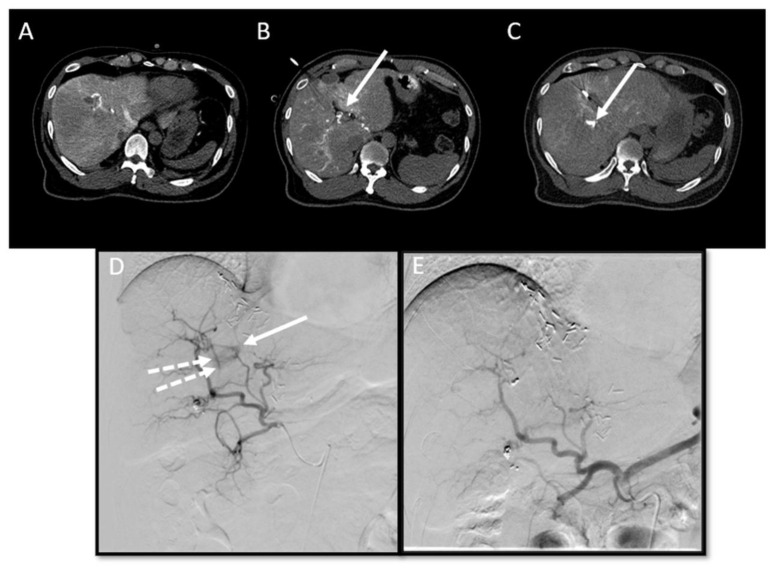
Critical care management: detection of post-ablation intraparenchymal hemorrhage and haemobilia. Probe-induced hepatic hemorrhage is seen in 0.7% of the patients [23]. Local tumor progression in segment 8 after parenchymal-sparing hepatectomy and intra-operative and percutaneous microwave ablations. After administration of 20 mL intra-arterial contrast (Visipaque™, iodixanol, 320 mg Iodine/mL, GE Healthcare Inc., Marlborough, MA, USA) in the common hepatic artery, the recurring tumor tissue became visible by a clear ‘incomplete ring sign’ adjacent to the prior ablation zone ((A), CTHA with a combined hybrid angio-CT system) [21]. Intraprocedural CTHA imaging depicted an incidental contrast agent within the bile duct (arrow), consistent with hemobilia (B). In addition, CTHA also depicted a small intraparenchymal contrast agent deposition (arrow), consistent with intraparenchymal hemorrhage (C). Immediately after the completion of ablation (Emprint™ Microwave Ablation System, Medtronic-Covidien, Boulder, CO, USA), digital subtraction angiography (DSA; contrast agent, Omnipaque 300, GE Healthcare, Cork, Ireland) of the common hepatic artery was performed, disclosing contrast pooling from the A4 branch ((D), arrow), as well as hemobilia ((D), dashed arrows), for which a combination of glue, Lipiodol^®^ Ultra Fluid contrast agent (Guerbet, Villepinte, France) and coils was used. Post-embolization DSA of the A4 and A8 branches depicted bleeding cessation (E).
